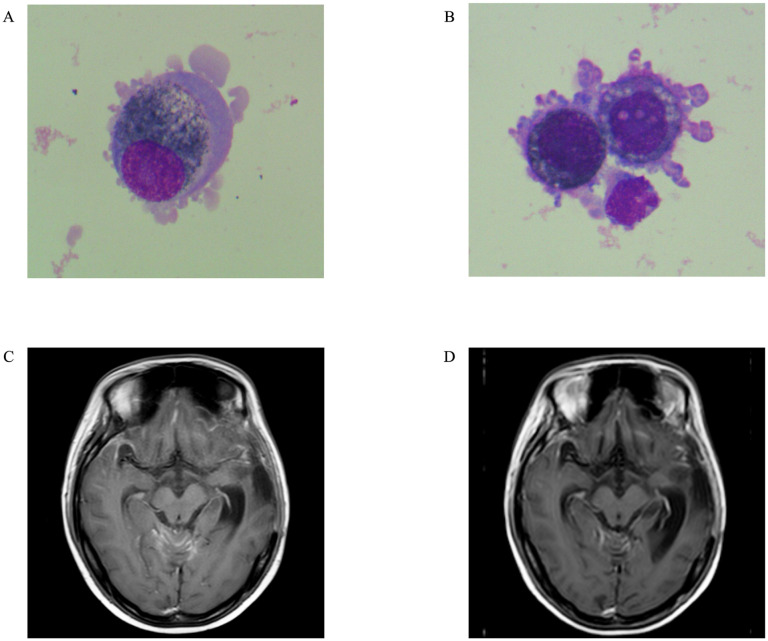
Cytopathologic and radiographic findings in a representative patient with melanoma leptomeningeal metastasis. Cerebrospinal fluid cytology demonstrating malignant melanoma cells before intrathecal anti-PD-1 treatment (A, B). MRI findings of patient 10 before intrathecal anti-PD-1 treatment (C) and reduction of leptomeningeal lesions (D).
